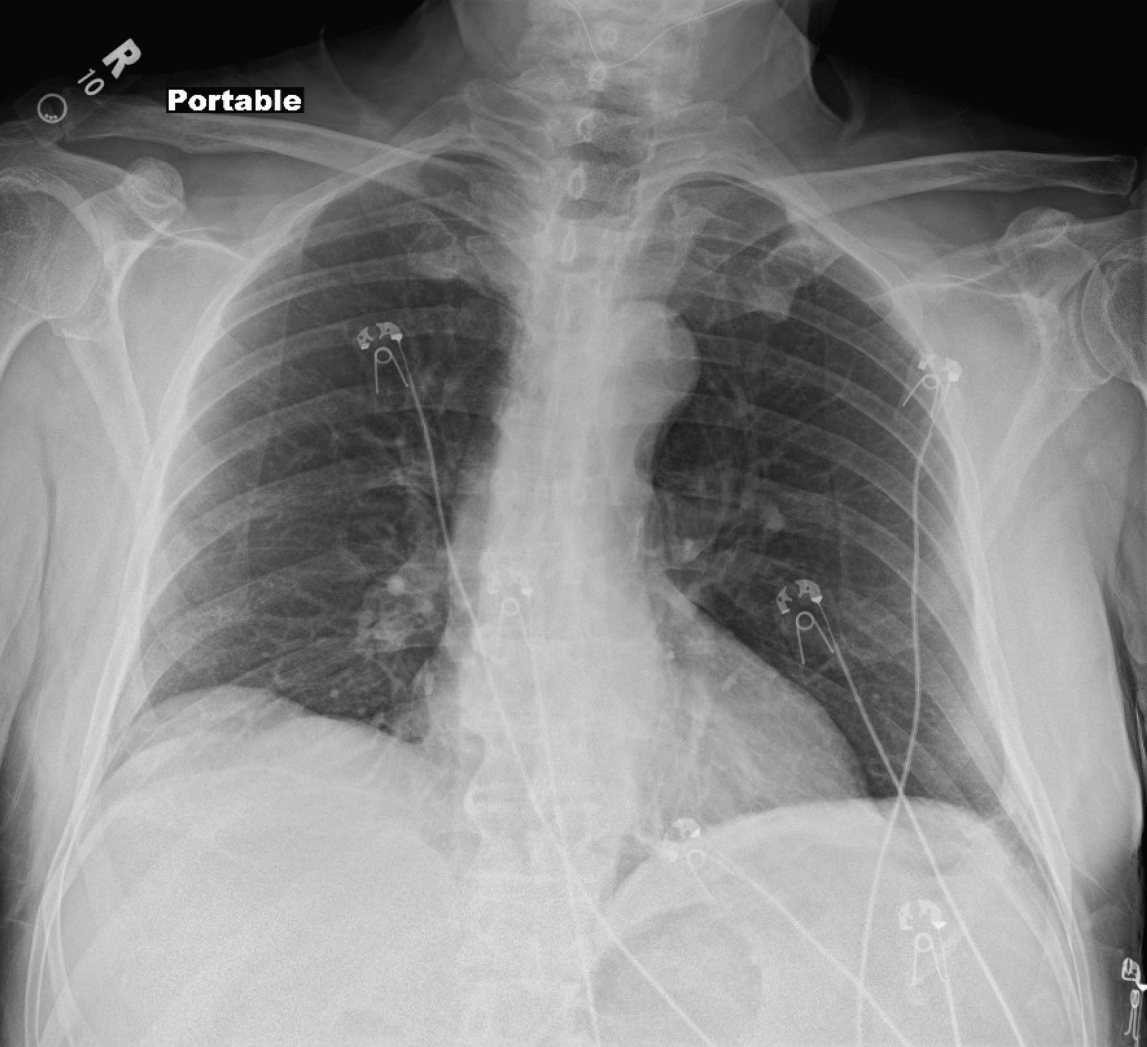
Chest radiograph showing mild right lower lobe scarring without focal consolidations.
